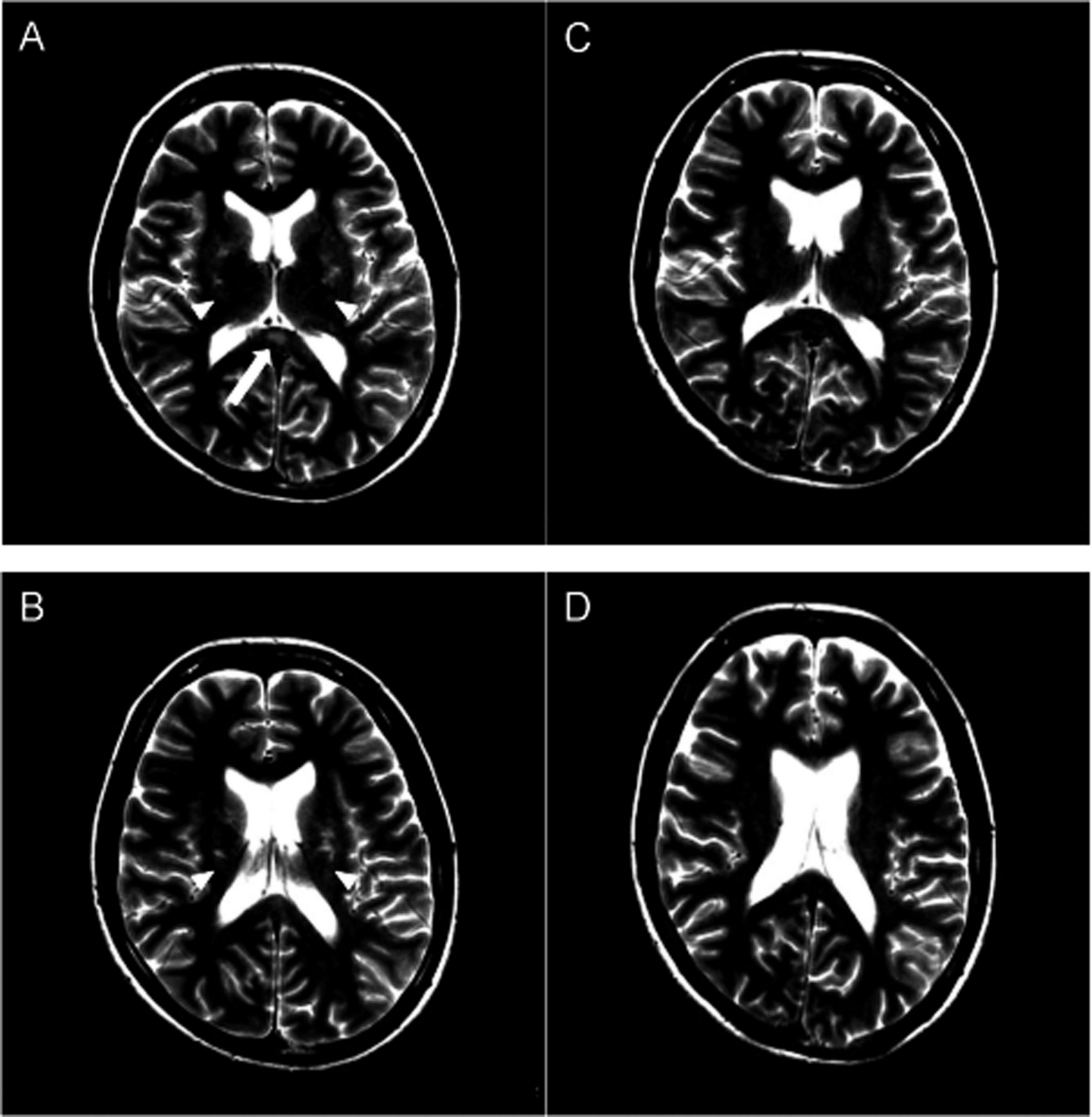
Mild encephalitis/encephalopathy with a reversible splenial lesion and white matter lesions. (A,B) T2-weighted images at day five showed a lesion with high signal intensity in the central portion of the splenium of the corpus callosum (SCC) and cerebral white matter lesions (arrow, SCC; arrowhead, cerebral white matter lesions). (C,D) Follow-up MRI scan on day 17 showed no lesions on any sequences (T2-weighted image).
